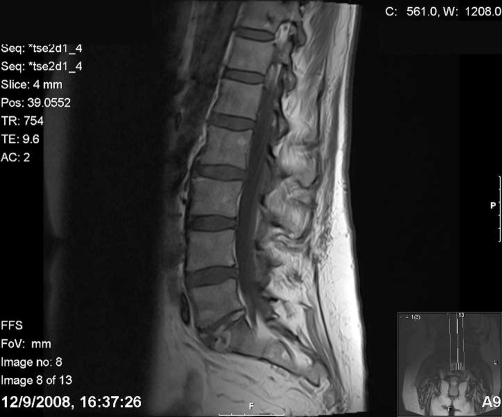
MRI Lumbar Spine showing spondylodiscitis at L5/S1 level.
